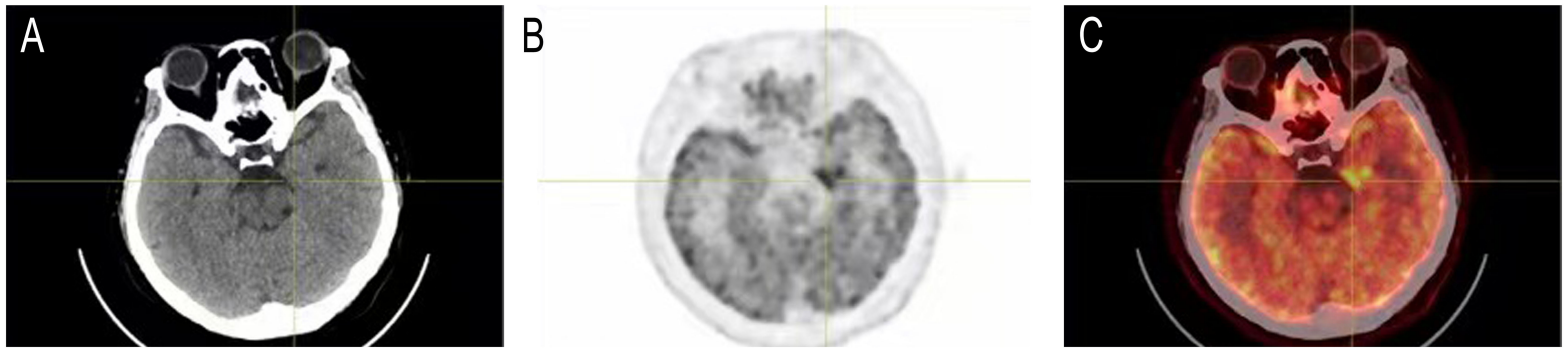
Head PET-CT of case 4. (A) CT imaging. (B) PET imaging. (C) Merged of CT and PET imaging showed concentrated radioactive distribution in the medial temporal lobe of the left side.
